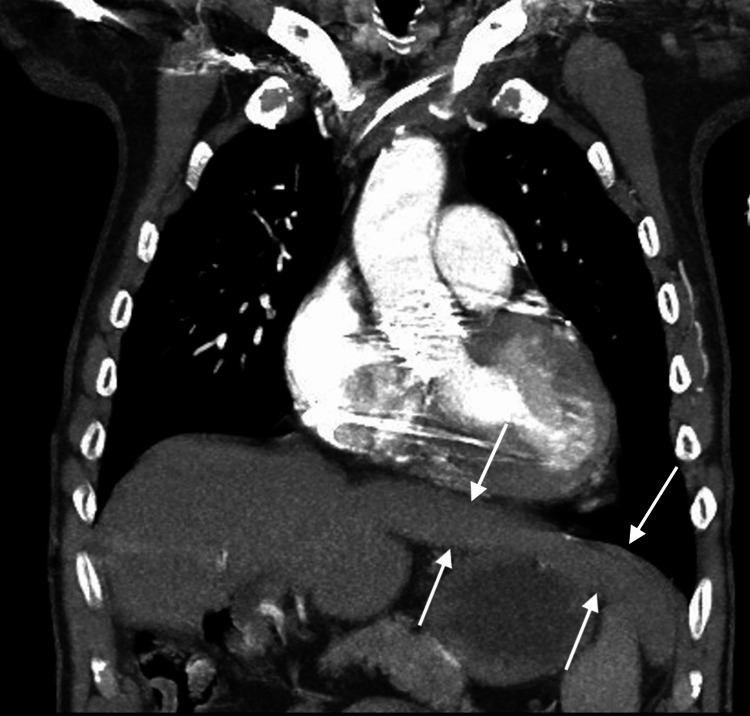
CT angiography of the chest reveals the incidental finding of the beaver tail liver as indicated by the arrows.
